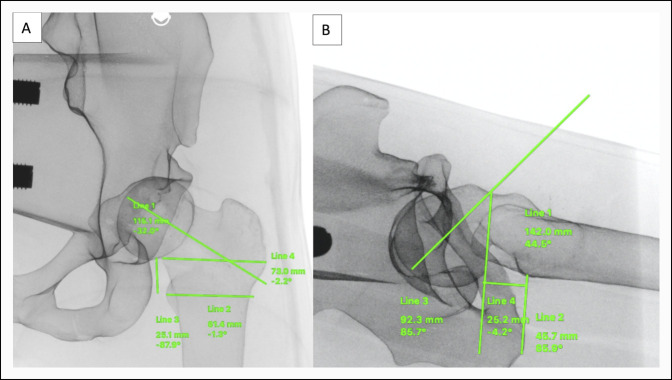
Radiographs showing surgical preoperative planning: participants were allowed to use preoperative planning software (Surgimap; Nemaris) to map out any planned screw trajectories on (A) AP and (B) lateral radiographs immediately before evaluation.
